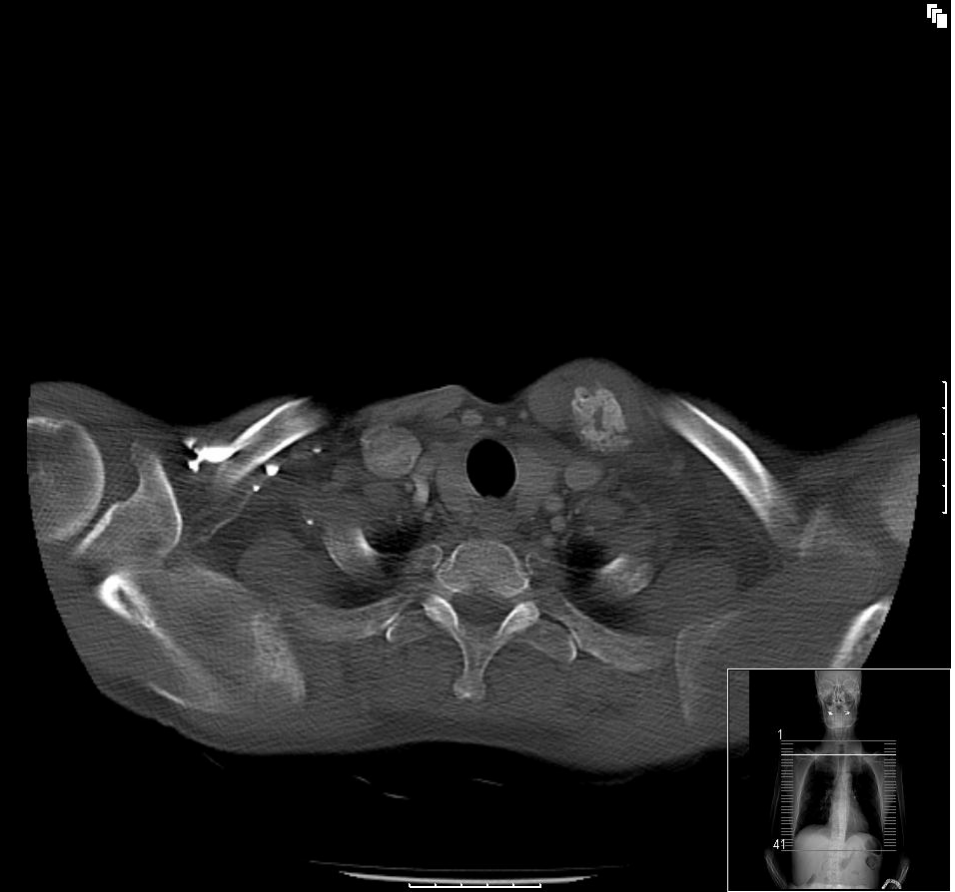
Axial CT image showing a well circumscribed osseous lesion within the sternocleidomastoid muscle above the site of the fracture (day 8).
